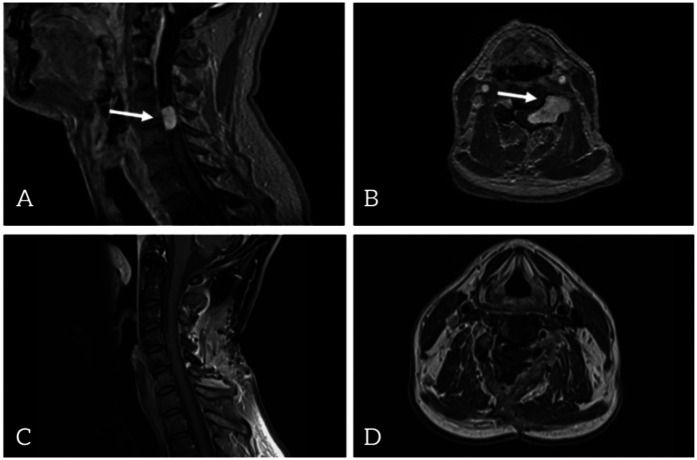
Contrast-enhanced T1-weighted magnetic resonance imaging (MRI) preoperative images: sagittal view (A) and axial view (B) postoperative images: sagittal view (C) and axial view (D).
